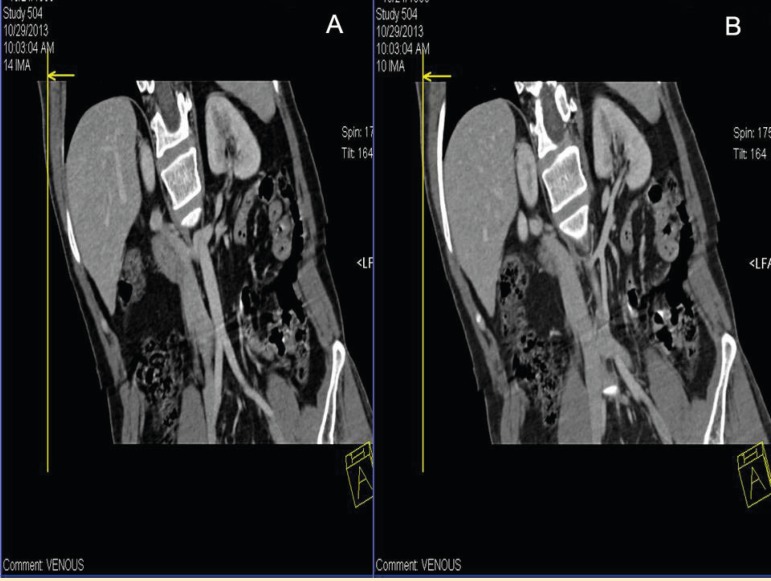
Coronal reformatted image of the venous phase of CT renal angiography showing two retro-aortic left renal vein with drainage into the IVC as well as the left common Iliac vein
